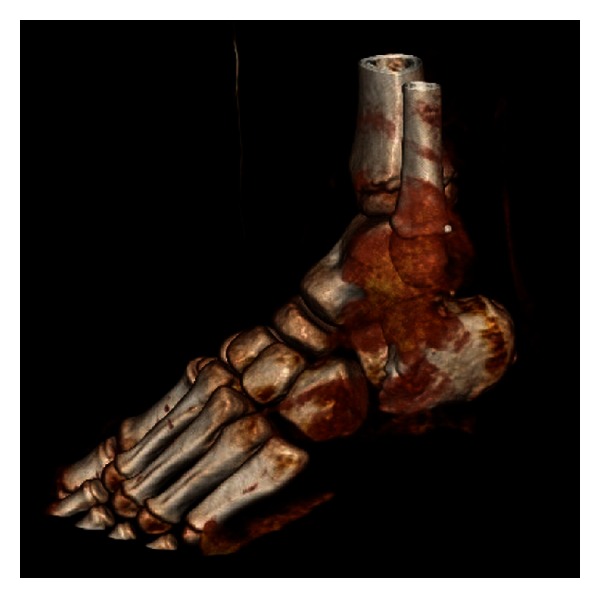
CT 3D reconstruction of left ankle displaying fracture and dislocation through calcaneal body.
